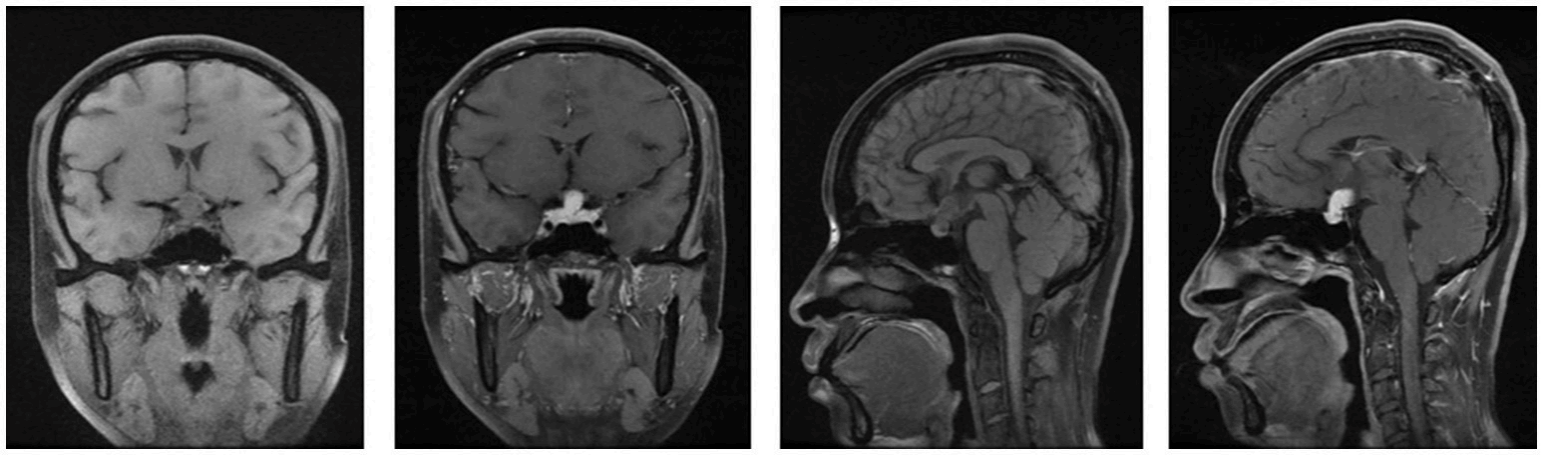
The MRI of brain and pituitary (second hospitalization). No signs of shrinkage for the mass.
